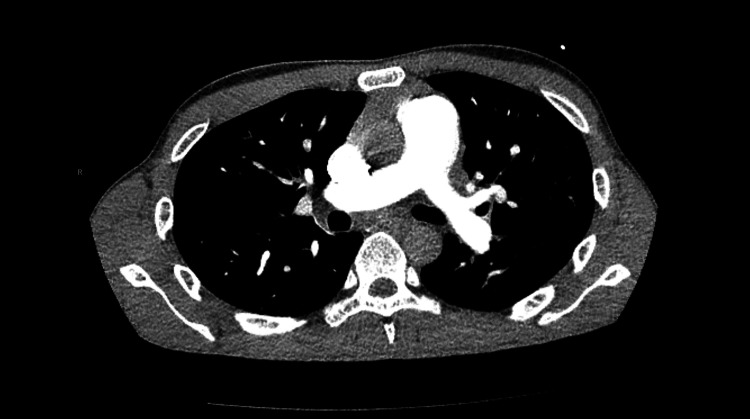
CT angiography of the chest negative for pulmonary embolism
